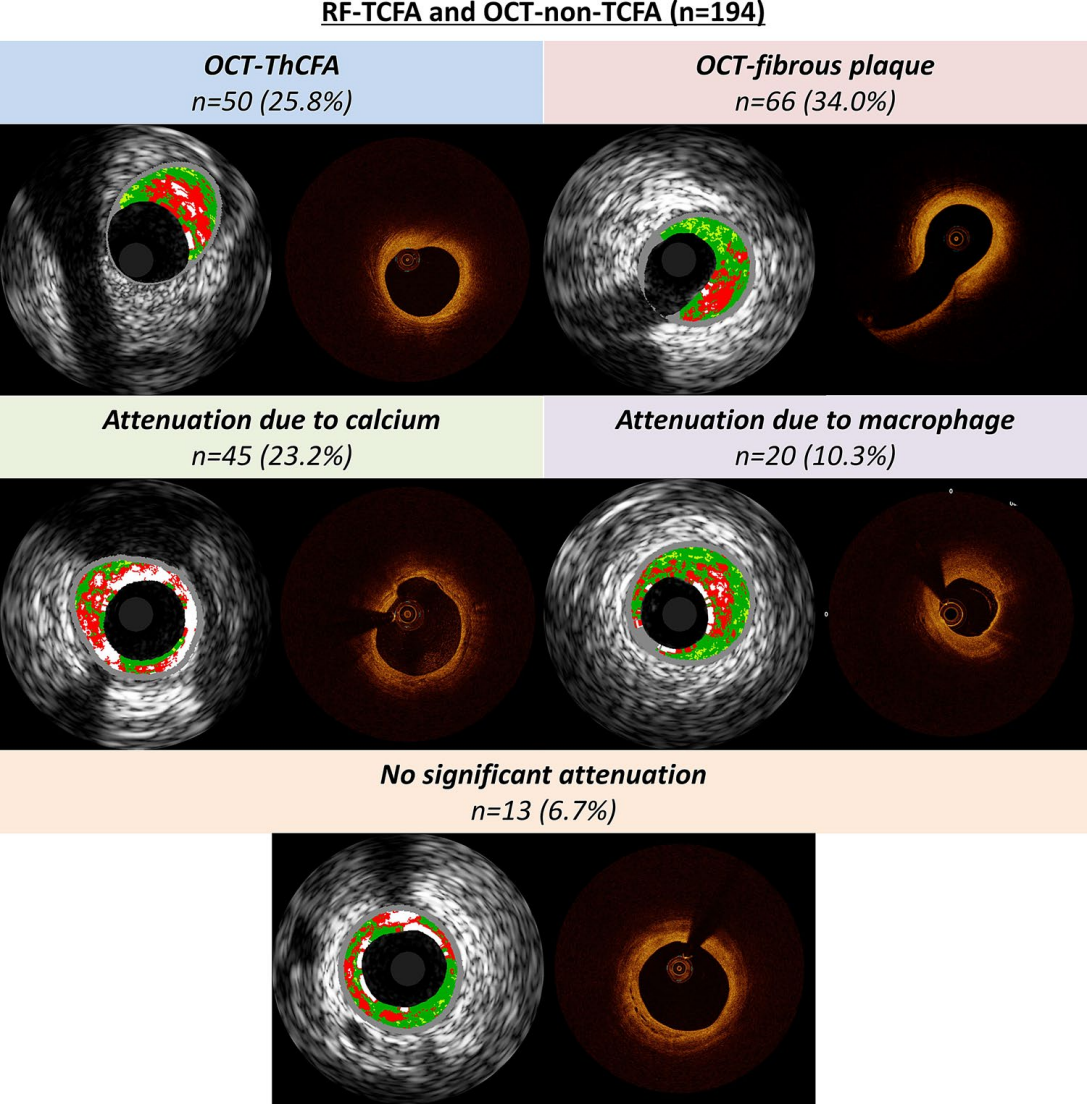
Principal reasons for discordance between RF-IVUS and OCT. *IVUS* intravascular ultrasound, *OCT* optical coherence tomography, *TCFA* thin-cap fibroatheroma, *ThCFA* thick-cap fibroatheroma, *RF* radiofrequency
